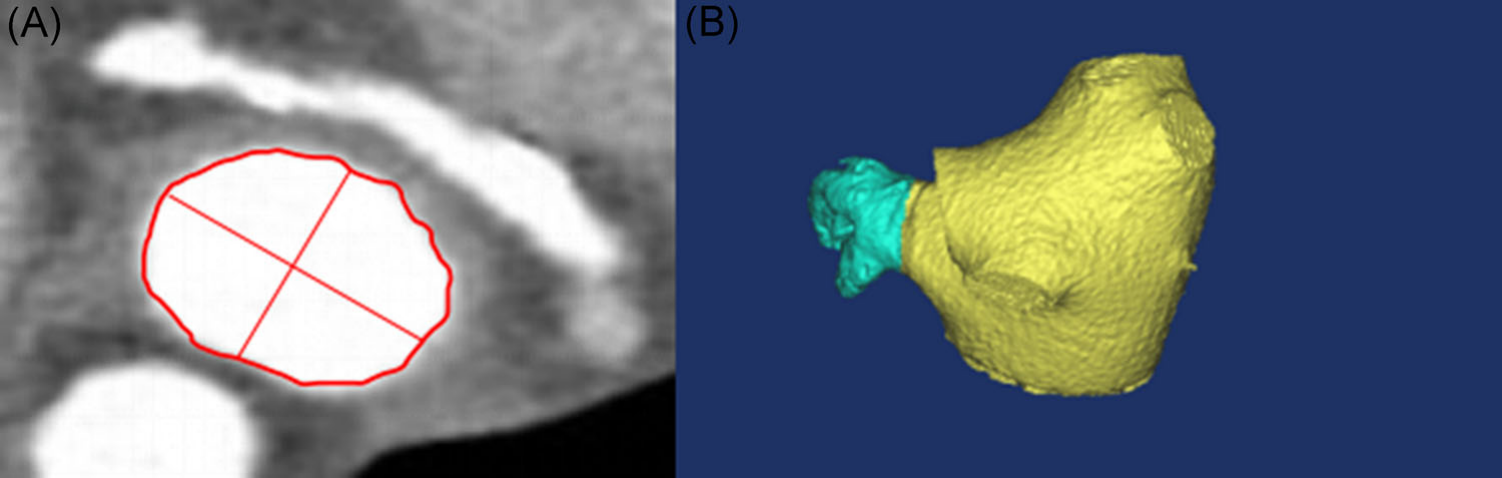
CCTA Images with multiplanar and three‐dimensional (3D) reconstruction of the LAA orifice. (A) The multiplanar reconstruction imaging of the LAA orifice, allowing to measure the maximum diameter. (B) The 3D reconstruction imaging of LA and LAA, LAA separation, LA and LAA volume calculation. CCTA, cardiac computed tomography angiography; LAA, left atrial appendage; LA, left atrium
